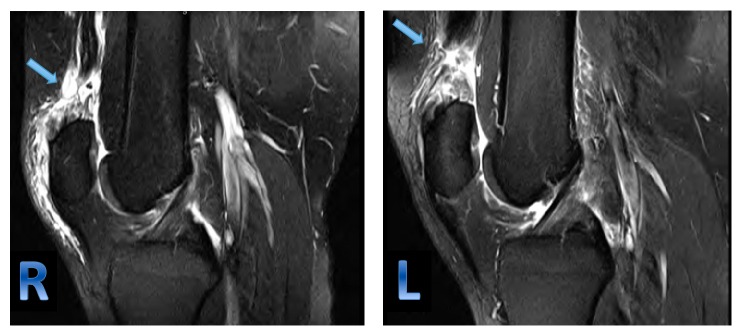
MRI of right and left knees showing T2-weighted sagittal image and demonstrating a full-thickness tear of the quadriceps tendon at the osteotendinous junction and fluid within the tendon gap with some retraction of the tendon which is more pronounced on the left side.
